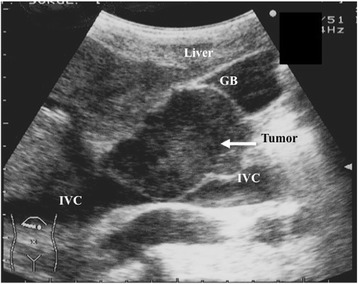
Abdominal ultrasonography. Abdominal ultrasonography revealed an abnormal mass in the infrahepatic portion, which compressed the gall bladder and liver. *GB* gall bladder, *IVC* inferior vena cava
